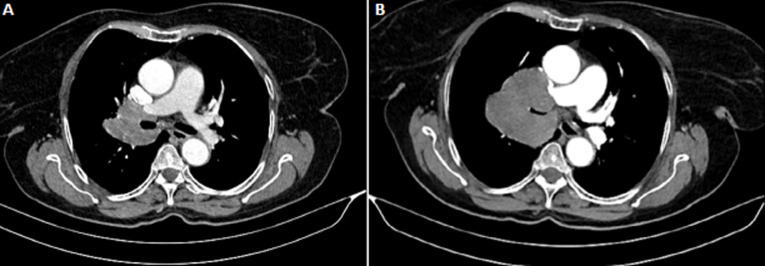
A) chest computed tomography (CT) shows a right upper lobe tissue process; B) chest computerized tomography (CT) done a year later showing an increase in the volume of the mass with compression of the bronchovascular structures
